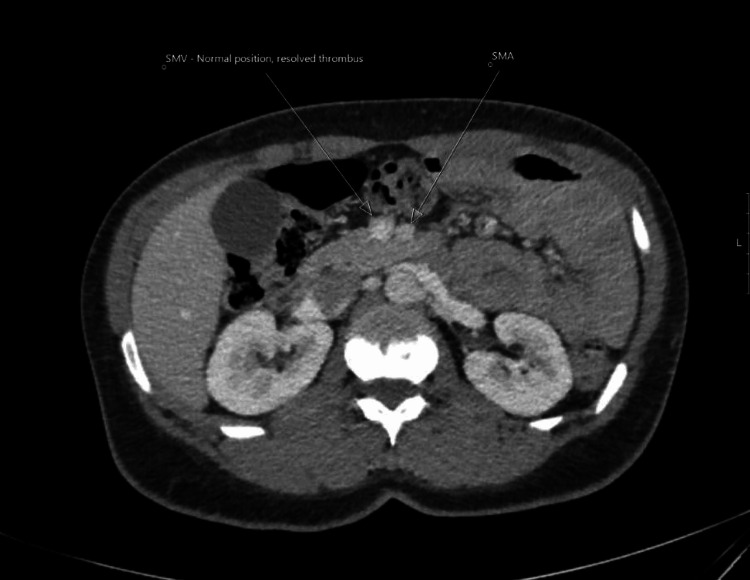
CT scan demonstrating normal SMV showing thrombus resolution (arrow) and SMA (arrow) CT: computed tomography, SMV: superior mesenteric vein, SMA: superior mesenteric artery
